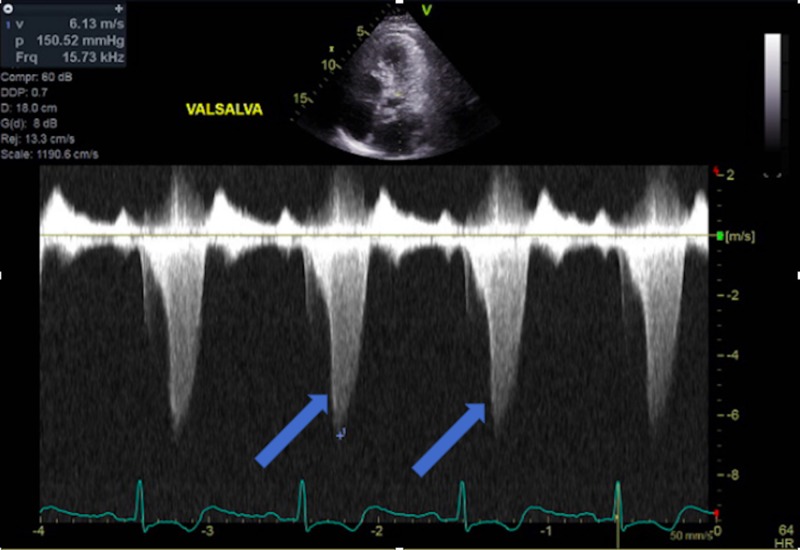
CW Doppler across LVOT with induced Valsalva showing an increased pressure gradient across LVOT CW Doppler: continuous Doppler; LVOT: left ventricular outflow tract
